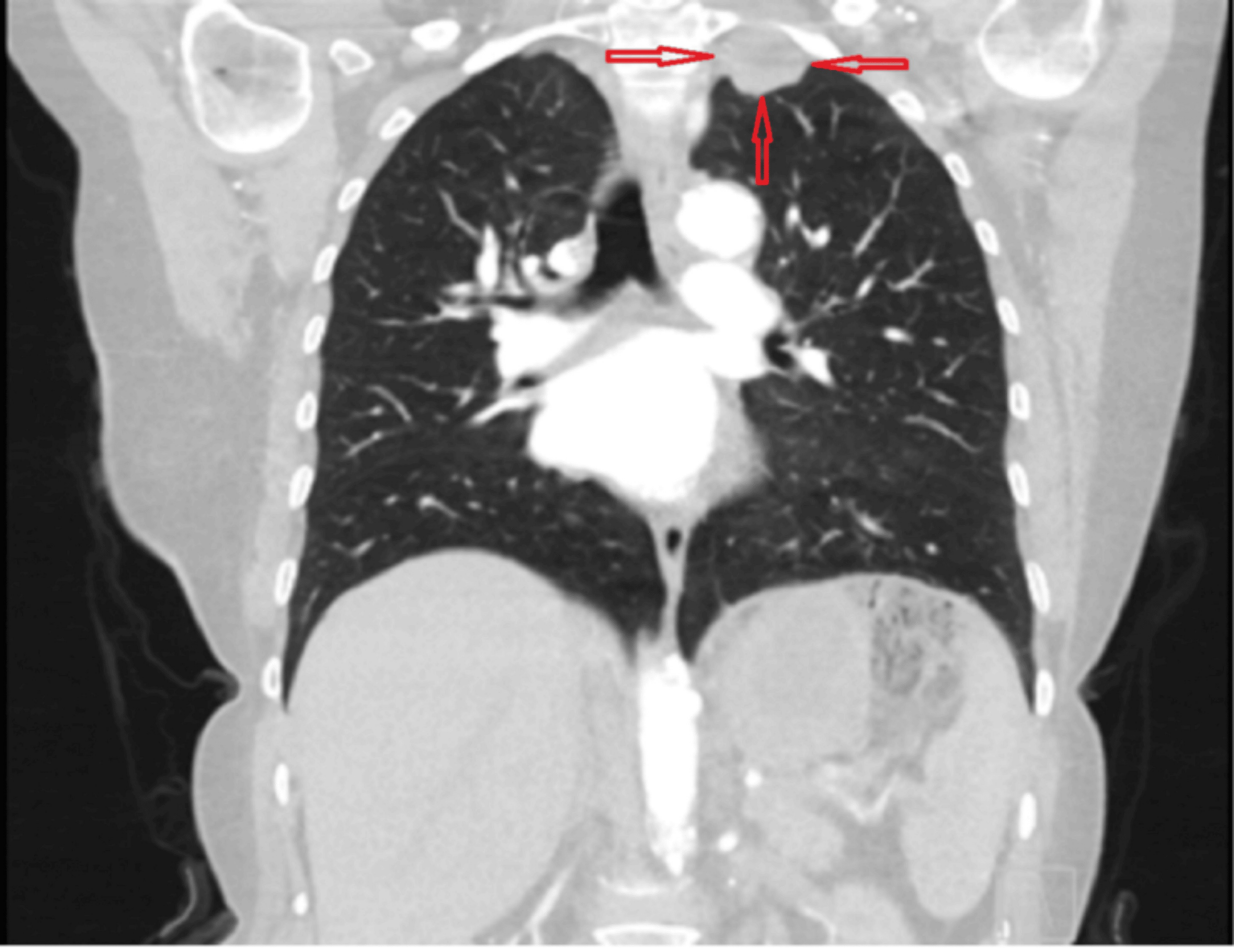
Coronal section of thoracic computed tomography showing the location and appearance of the lesion.
